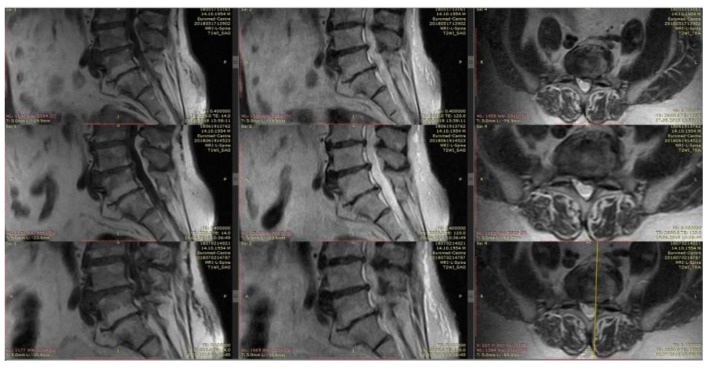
MRI of the lumbar spine images show a large extruded disc fragment at the L5-S1 level. Neuroimaging for 15 and 30 days does not indicate changes of the size of extrusion.
